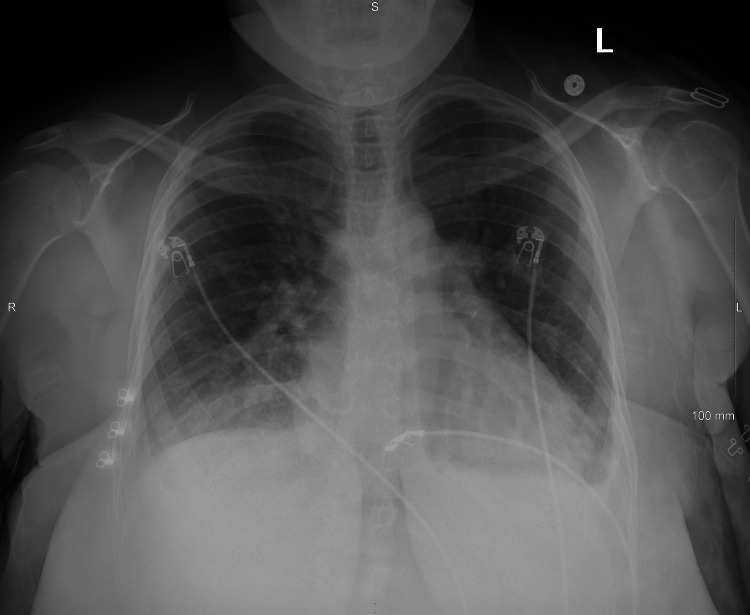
Chest X-ray demonstrating mild pulmonary edema with minimal bilateral pleural effusion
